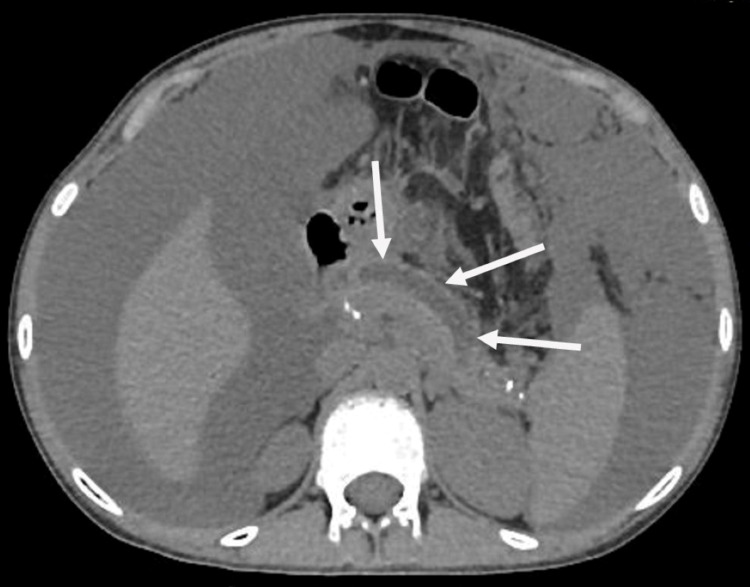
CT abdomen showing pancreatic inflammation and edema (white arrows). CT: computed tomography
